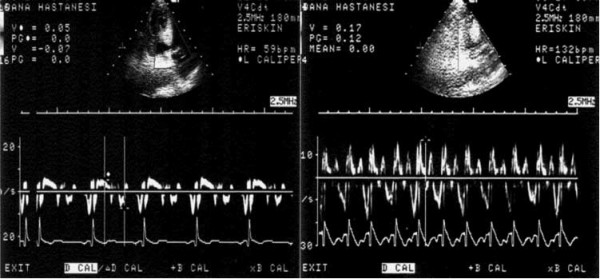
Dobutamine stress echocardiographic images. Measurements of maximum basal septal tissue systolic velocities at rest and peak stress in a hypertensive patient with basal septal hypertrophy [30].
